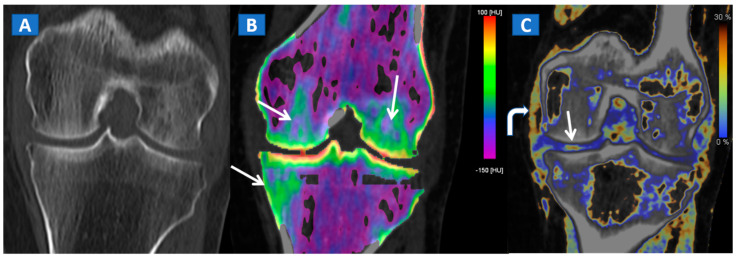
A 65-year-old female with a post-traumatic medial menisci rupture and diffuse BME. On the standard 1 mm reconstructed coronal CT image (A), there are no signs of fracture. On the corresponding 2D 1 mm reconstructed 2D DECT image (B), the BME appears more pronounced on the femoral condyles and on the medial tibial plateau (arrows). By using different color-coding (C), it was possible to identify a rupture of the medial menisci (straight arrow), with thickening and oedema of the medial collateral ligaments (curved arrow).
